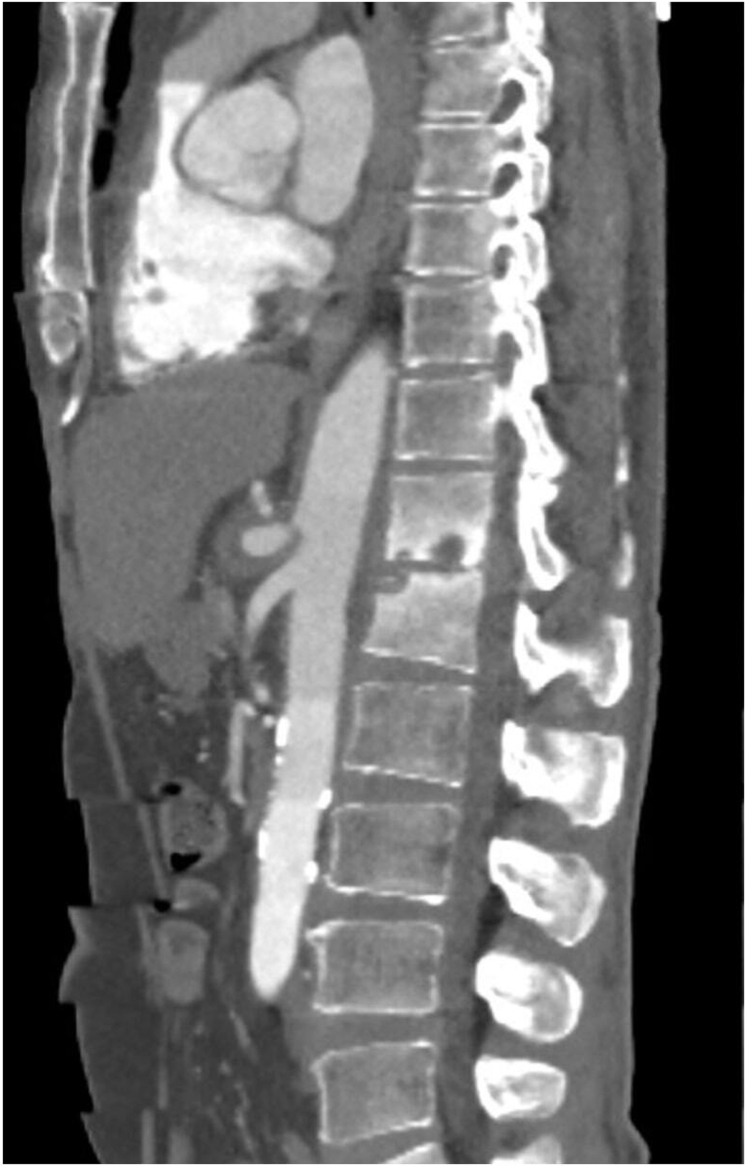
Computed tomography angiography (CTA) displaying sagittal view of focal aneurysmal dilatation of the proximal celiac trunk, ≤1.1 cm, with subsequent total occlusion of the celiac artery ∼2 cm from its ostium.
